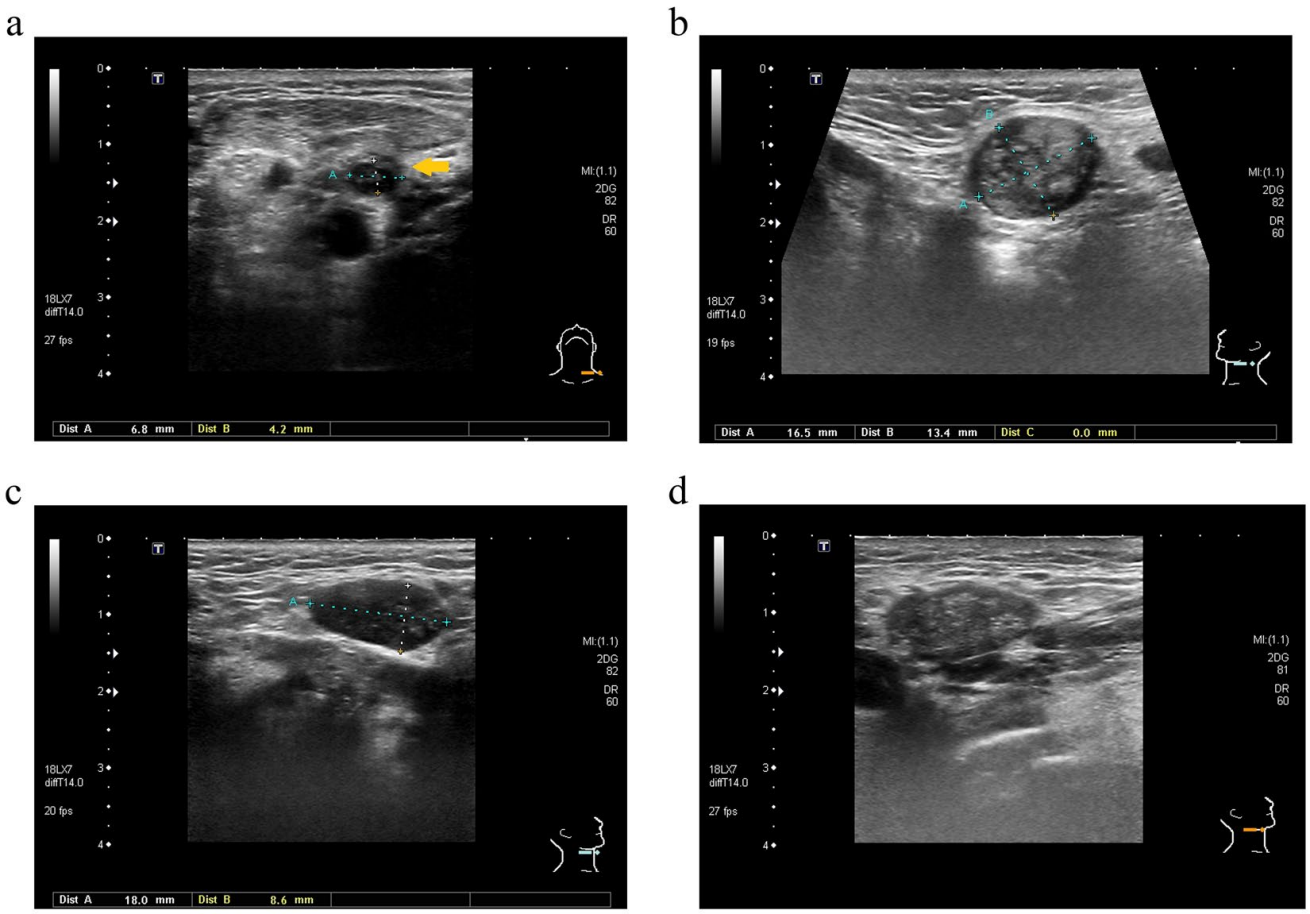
All significant ultrasound features of malignant lymph nodes (LNs) in the treated neck of head and neck cancer patients: (a) Left level IV recurrent LN with irregular margin (interrupted margin) (arrow), malignant (b) Left level IIa recurrent LN with round shape (short/long axis ratio >0.5), malignant (c) Right level Ib recurrent LN with size >0.8 cm, short axis, malignant (d) Right level Ib recurrent LN with heterogeneous internal echo, malignant.
